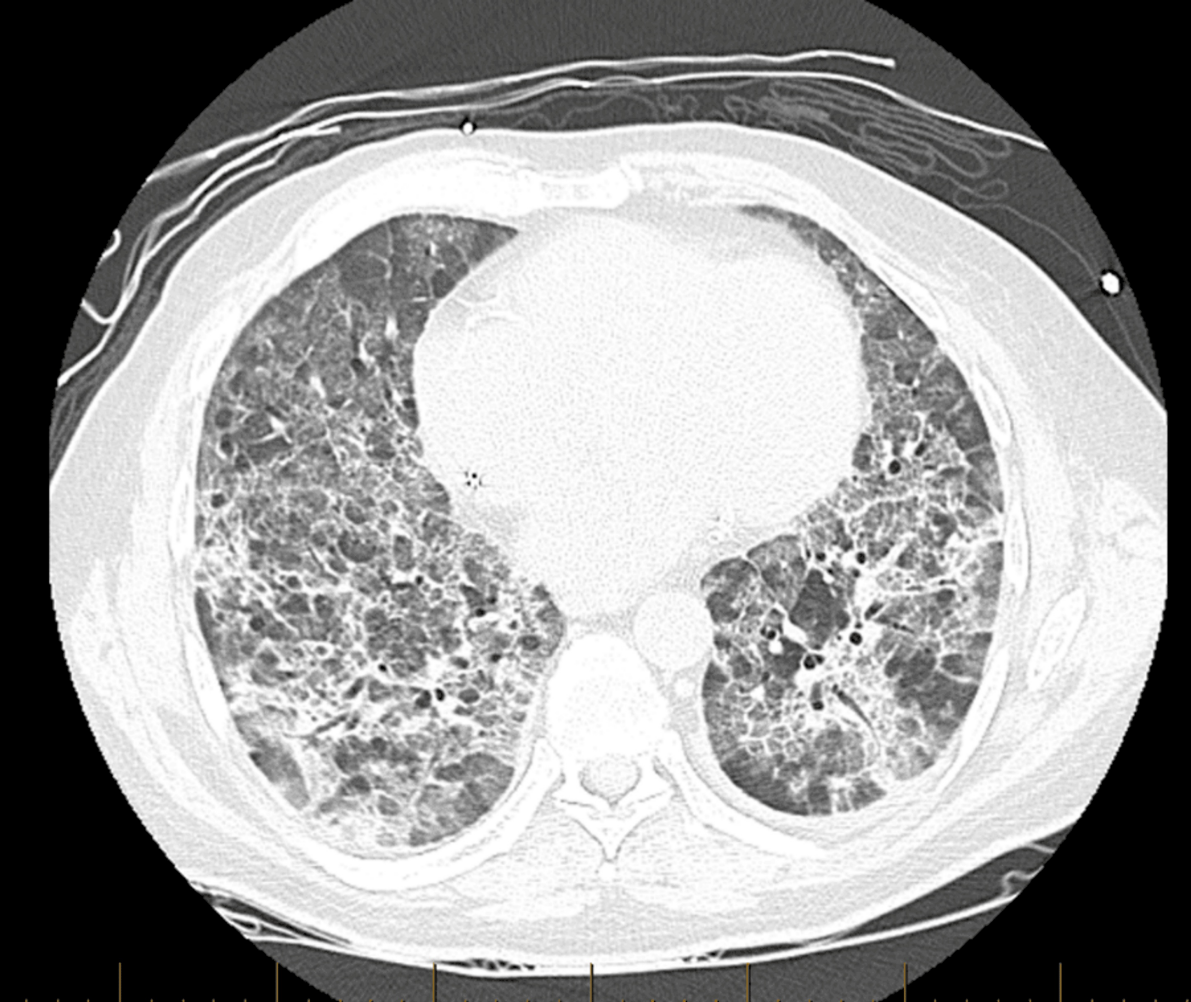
Axial non-contrast high-resolution computed tomography (HRCT) of the chest performed one week into hospitalization, demonstrating worsening lower lobe opacities, interlobular septal thickening, and new mild cylindrical bronchiectasis, with findings suggestive of possible early fibrosis
